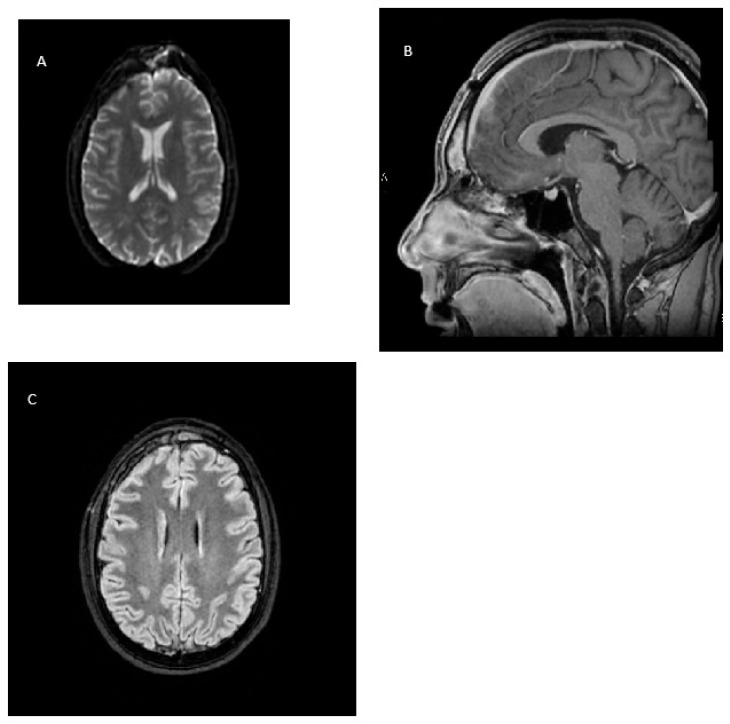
Magnetic resonance follow-up 40 days after surgical drainage and targeted antibiotic therapy. (A) DWI sequence reveals no evidence of restricted diffusion, confirming resolution of the abscess cavity. (B) 3D T1-weighted gradient echo demonstrates complete resolution of the previously observed ring-enhancing lesion in the right frontal lobe. (C) Axial FLAIR image shows normalization of signal intensity in the affected region, with no residual edema.
